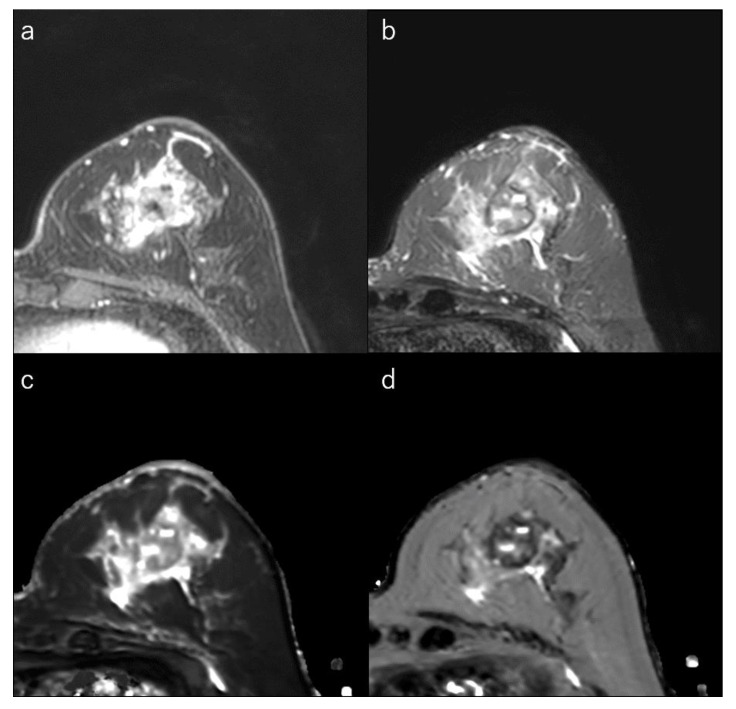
(a) Dynamic contrast-enhanced MR image in a 73-year-old woman with triple-negative breast cancer shows an irregular mass with rim enhancement. (b) Short-tau inversion recovery image shows focal areas of very high signal within the mass. (c) T1 map (window width/centre = 1400/2400 ms) shows focal very high signals; Mean T1 of the mass is 1533 ms. (d) T2 map (window width/centre = 160/240 ms) shows focal very high signals within the mass; and mean T2 of the mass is 113 ms.
